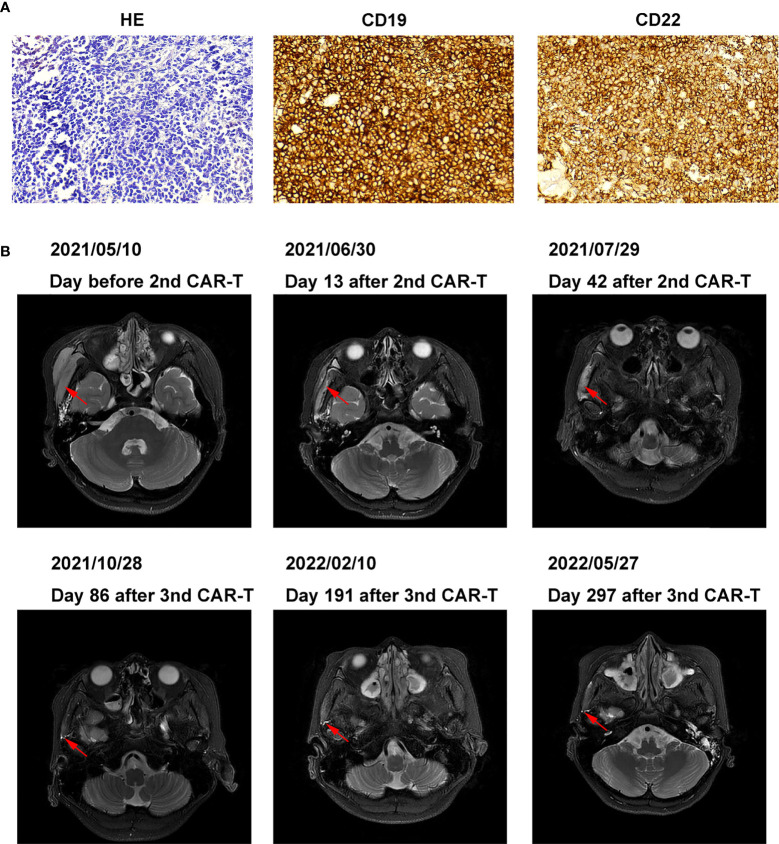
Pathology, histochemistry, and MRI images of right facial mass (A) Pathology and histochemistry of right facial mass. (B) MRI images of the right facial mass before and after 2nd, the 3rd CAR-T cell treatment.
